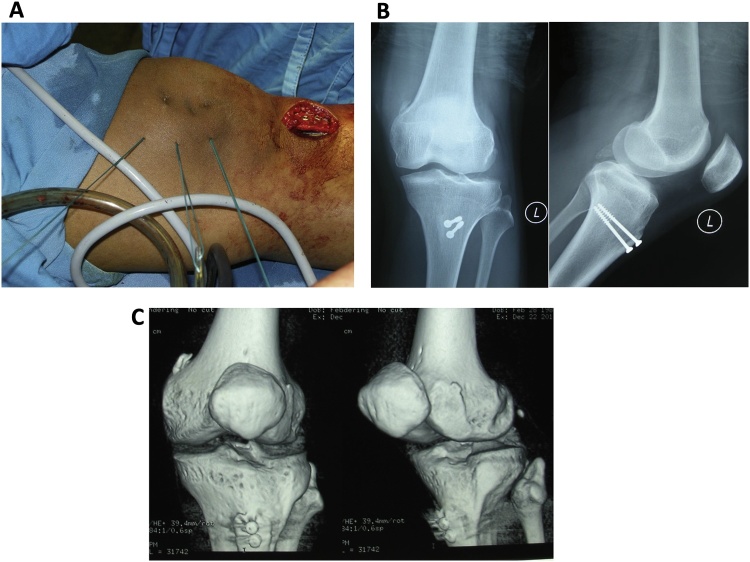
A. Percutaenous medial plication using non-absorbable string no.2. B. Post-operative anteroposterior and lateral projection of plain radiograph imaging. C. Post-operative CT scan.
